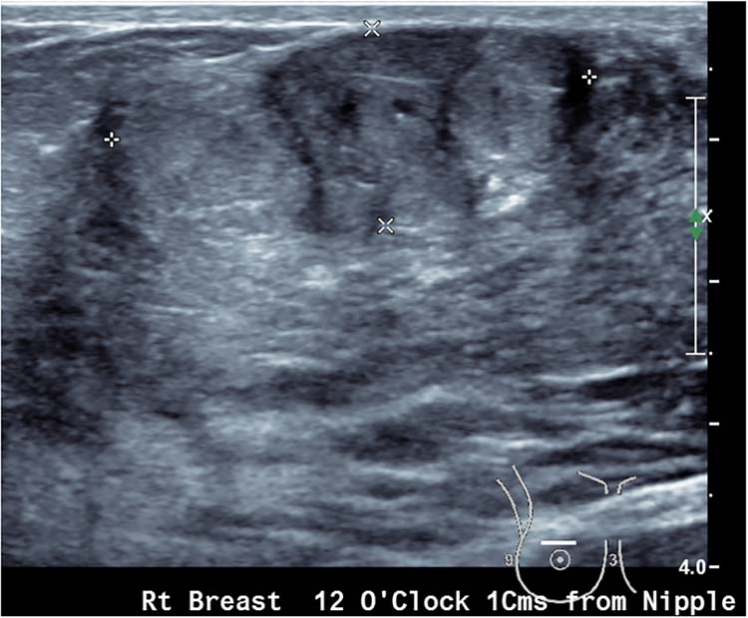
Ultrasound image showing a lobulated ovoid breast lesion.
